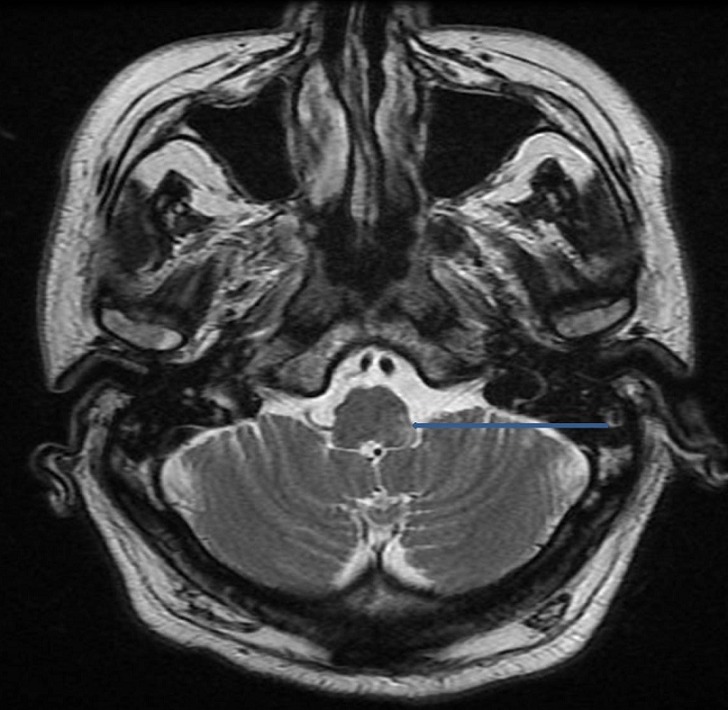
Cranial MRI showing a hyperintense lesion on T2 sequences in the right lateral medullary region
